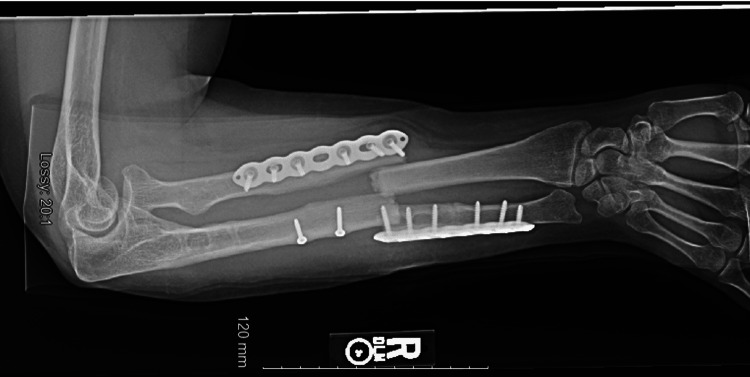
Anteroposterior forearm radiograph at the time of the injury. Independent screws and sclerosis can be seen in the metaphysis and proximal diaphysis of the ulna from her first fracture.
